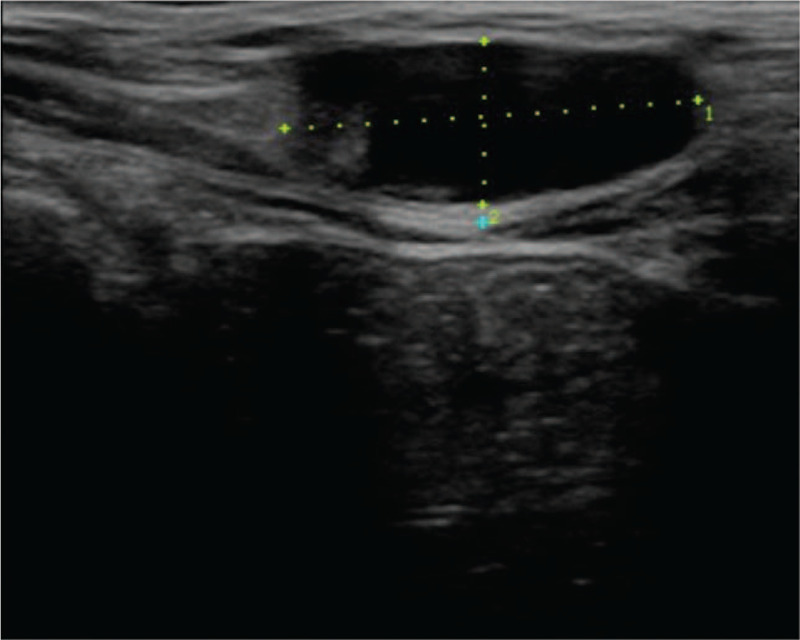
Ultrasound image of the pubic endometrioma showing a hypoechoic mass measuring 3.0 cm × 2.0 cm in the pubic region.
